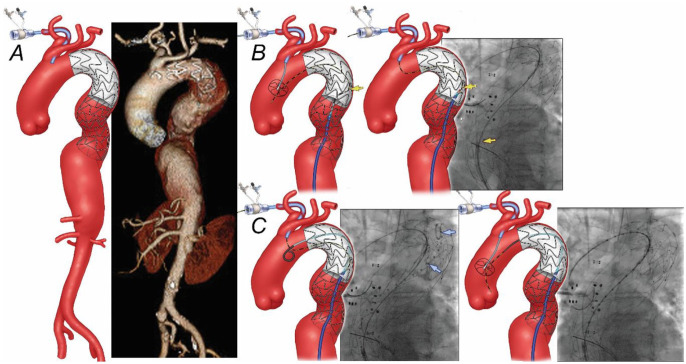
(A) Preoperative 3-dimensional computed tomography angiography reconstruction shows a 6.2 cm extent II thoracoabdominal aortic aneurysm distal to previous thoracic stent-graft and bare-metal dissection stent. (B) Snaring of a brachial-to-femoral guidewire was performed using a Kumpe catheter, which unfortunately was routed beneath one of the stent struts of the dissection stent (*yellow arrow*). (C) Once this issue was recognized, a “buddy” pigtail catheter was advanced proximally into the stent-graft (*blue arrow*), followed by redo snaring in the ascending aorta.
